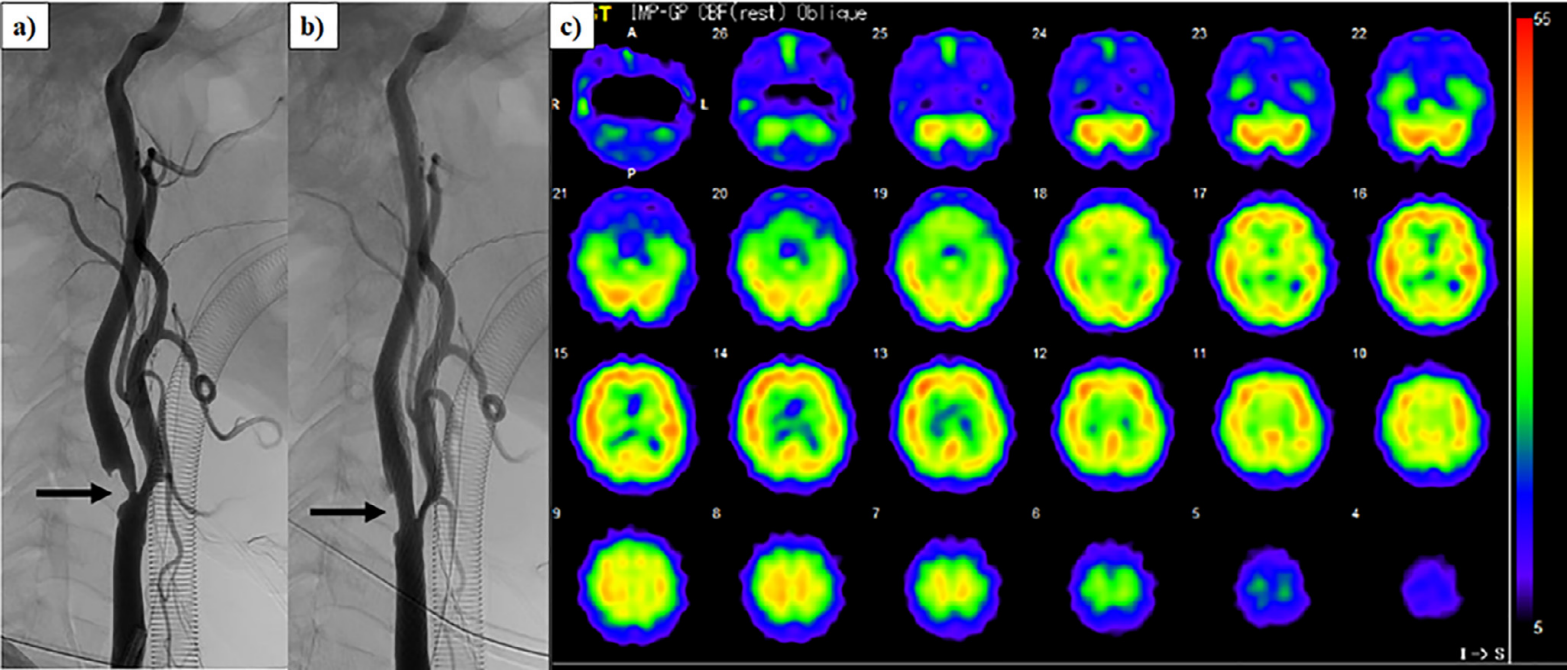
a) Severe stenosis of right internal carotid artery (arrow) demonstrated by cerebral angiography. b) Improvement of stenotic lesion of the right internal carotid artery (arrow) after carotid artery stenting. c)^123^I-N-isopropyl-p-iodoamphetamine single-photon emission computed tomography demonstrating no decreases in right cerebral blood flow.
